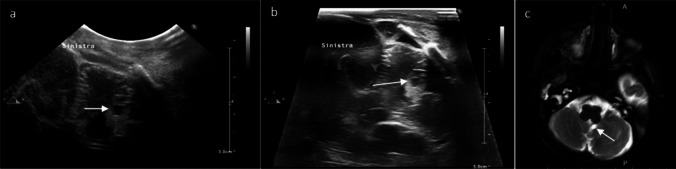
Cerebellar cystic lesion in a moderately preterm infant (gestational age 30 weeks), smaller twin born after dichorionic diamniotic pregnancy complicated by birth weight discordance. a, b Day 1 of life: coronal ultrasound scan using the left mastoid fontanel as acoustic window. Loss of parenchymal tissue of cerebellar vermis (arrow) is visible with the micro-convex transducer (a). Using the very high-frequency transducer (b), the echogenicity of the cerebellar parenchyma surrounding the cyst appears abnormally increased, raising suspicion of a prenatal cerebellar lesion with cystic evolution. MRI at term equivalent age (T1-weighted sequence, axial plane at the level of the brainstem) confirmed the cystic lesion (arrow), although its etiology remained undefined
